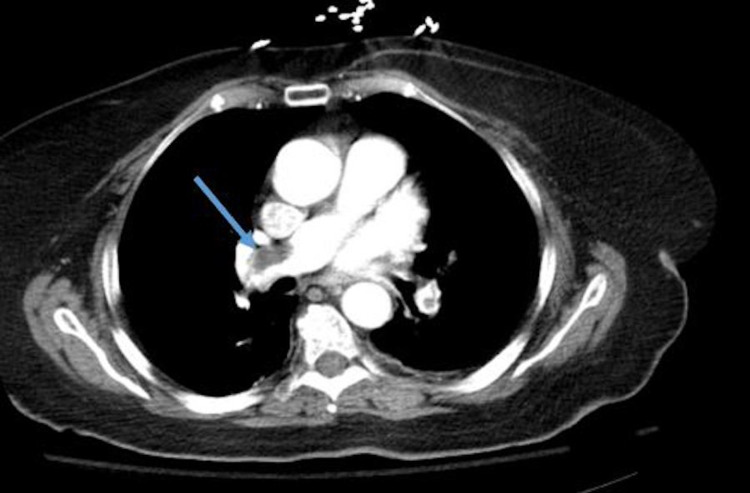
Computed tomography angiography (CTA) - blue arrow showing right pulmonary embolism
